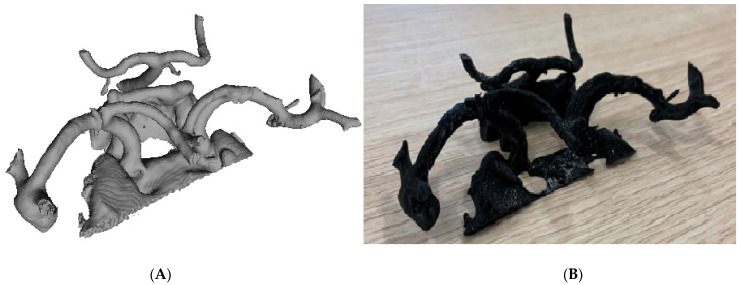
(A) STL file derived from CT-Angiography; (B) final 3D-model.
